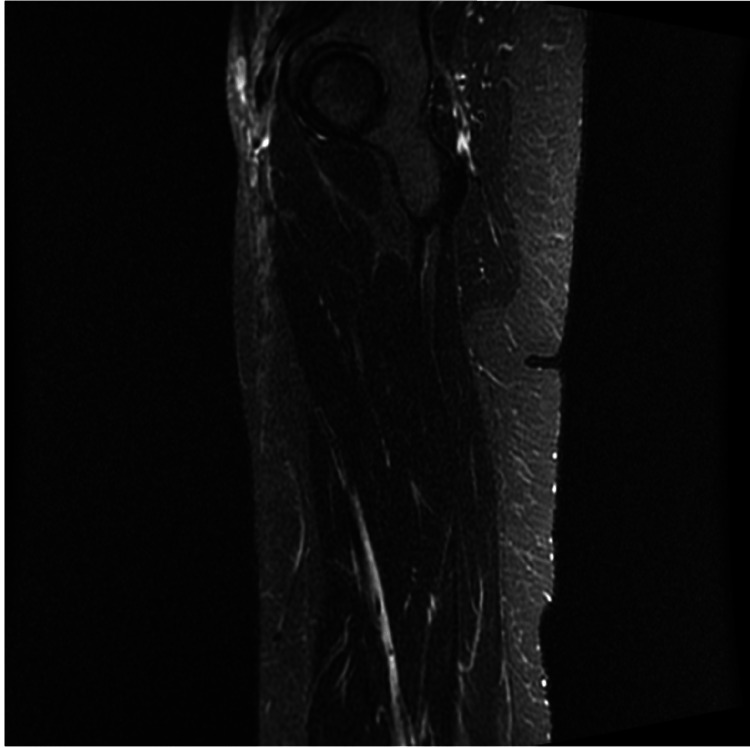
MRI of the left thigh. A, coronal T2-weighted (short tau inversion recovery) imaging showing normal muscle mass with no atrophy or edema
